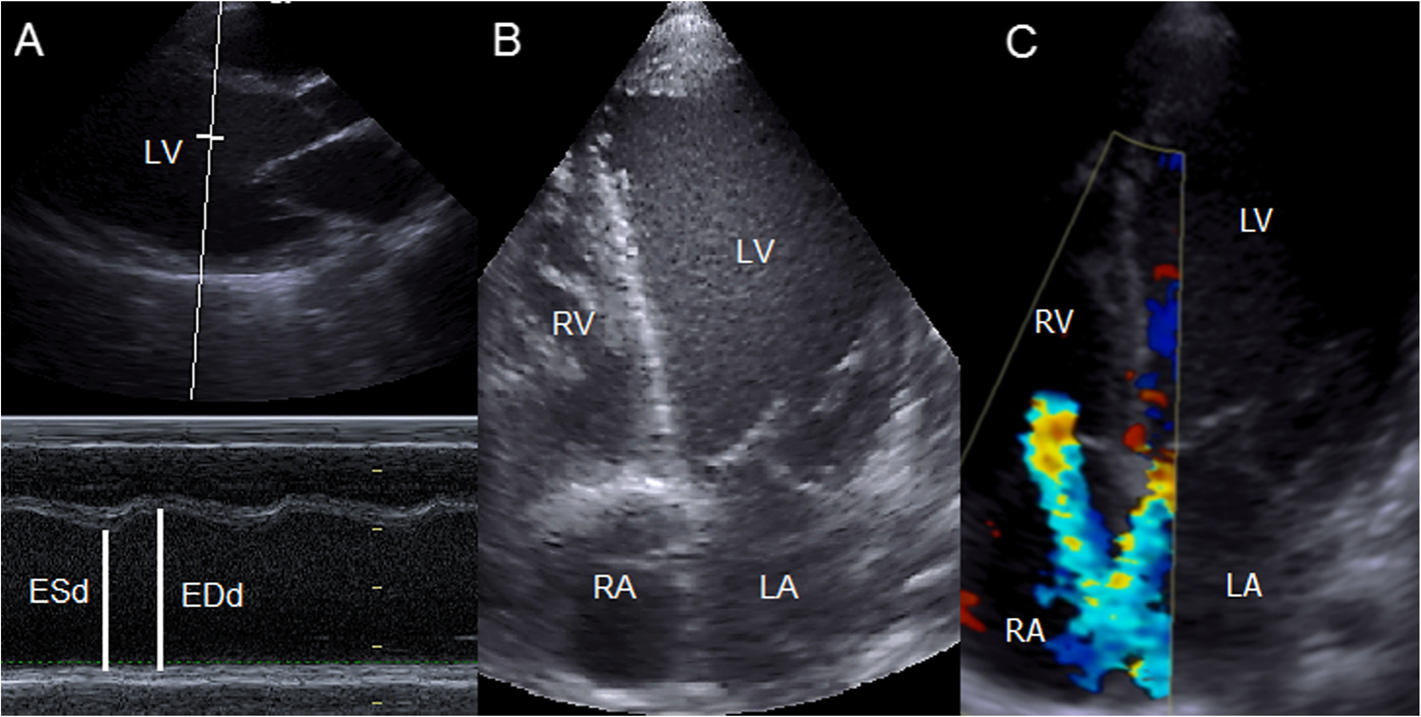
Four-year-old patient with important LV dysfunction. A- EF of 21% by M-mode parasternal long-axis view. B- Significant LV dilation and C- moderate TR seen from the apical 4-chamber view. EDd, end-diastolic diameter; ESd, end-systolic diameter; LA, left atrium; LV, left ventricle; RA, right atrium; RV, right ventricle.
